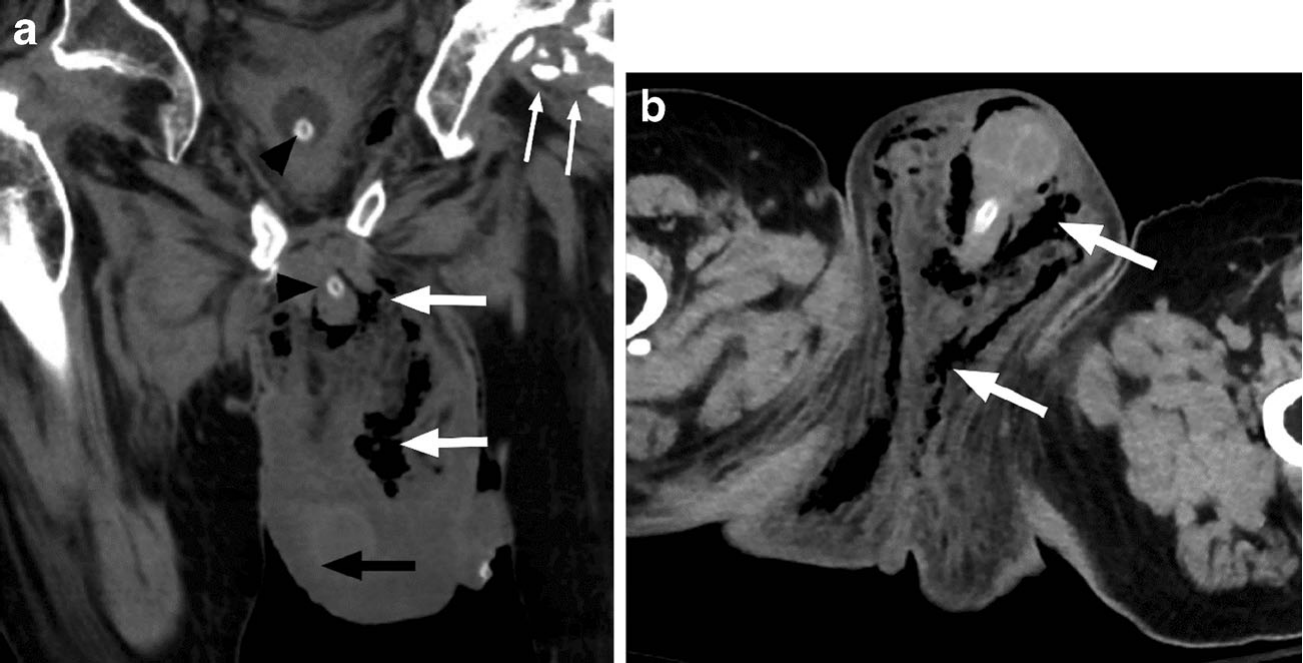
Fournier’s gangrene. a Coronal non-contrast CT image shows scrotal wall oedema (*black arrow*), with extensive subcutaneous gas in the scrotum and perineum (*white arrows*). Also note the presence of a bladder catheter (*black arrowheads*) and heterotopic ossification around the left hip (*small white arrows*). b Axial image from the same patient demonstrating the extent of infection and presence of gas throughout the perineum and scrotum (*white arrows*)
